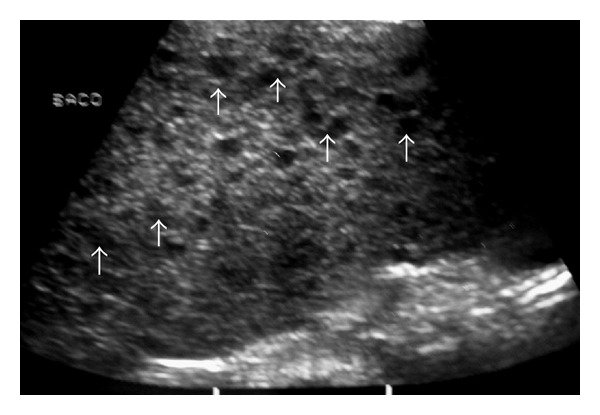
Young man with NHL. Sonogram of the spleen shows multiple hypoechoic nodules (arrows).
